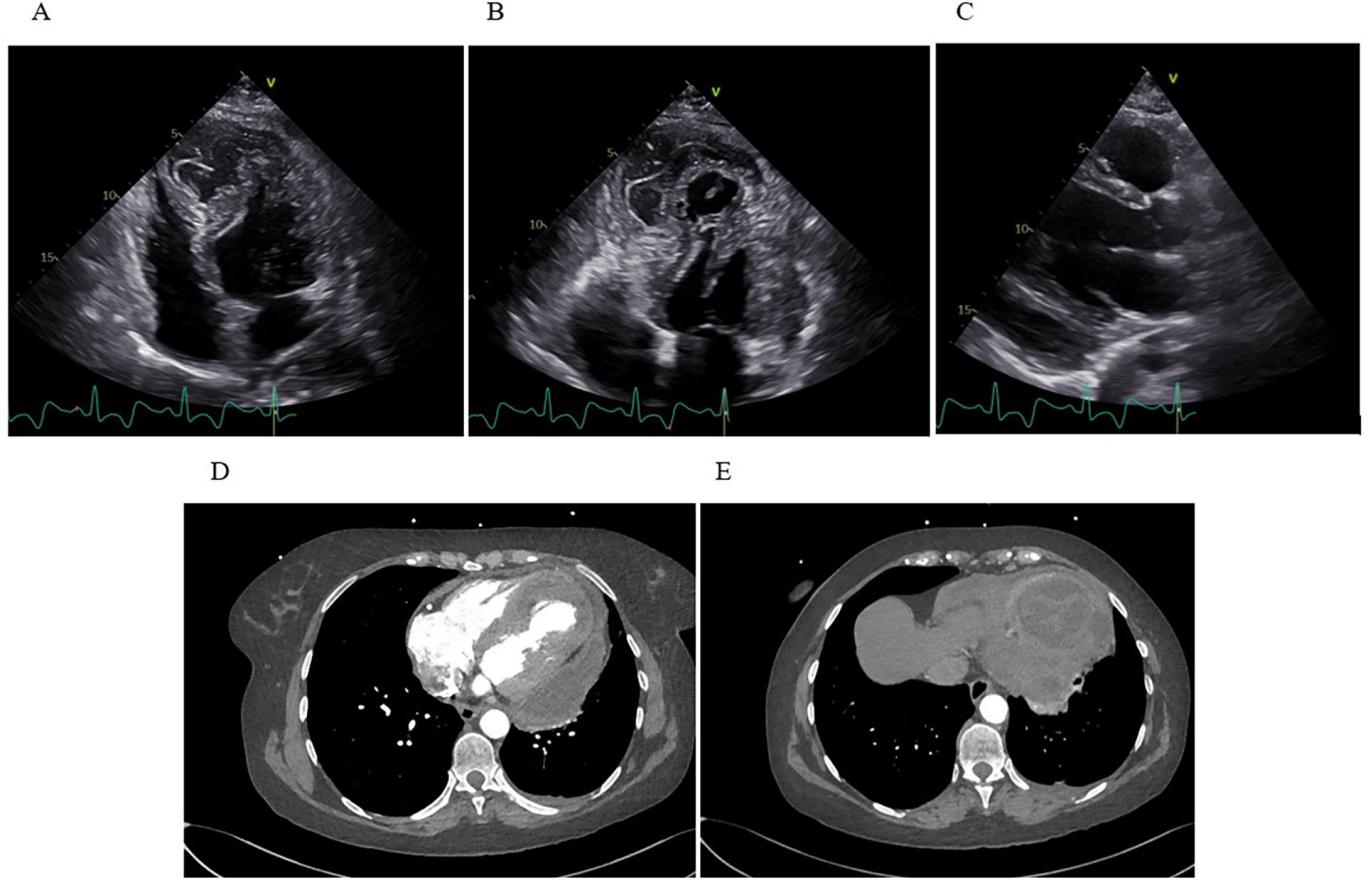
Transthoracic echocardiography and computed tomography. (A) Apical four-chamber view showing extensive IDH with marked apical dyskinesia. (B) Apical thrombus formation visualized as an echogenic mass at the ventricular apex. (C) The parasternal long-axis view reveals a moderate, new pericardial effusion along the inferolateral wall of the LV. (D) Axial CT demonstrates a multiloculated, heterogeneous fluid collection within the inferoapical myocardium, with clear communication to a large pericardial effusion along the lateral and posterior walls of the LV. (E) A separate axial CT slice shows a clearly defined, round, intramyocardial lesion.
